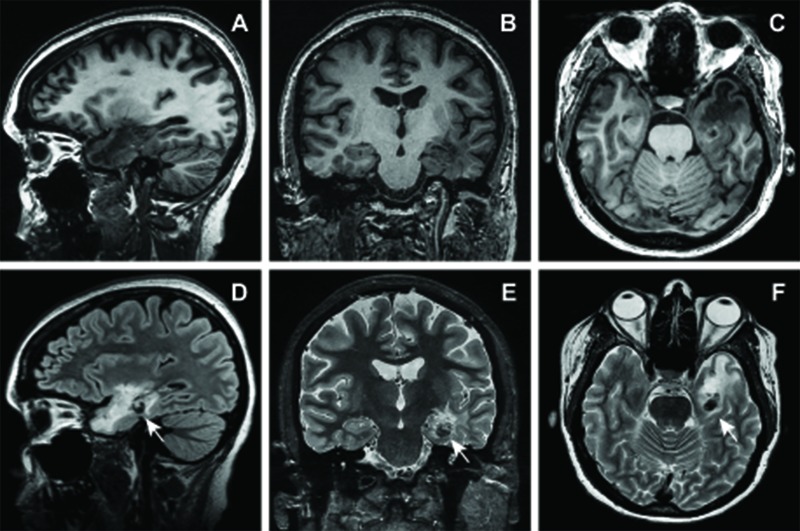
MRI obtained 9.5 years following radiosurgery showing suspected radiation-induced lesion. Sagittal, coronal and axial T1-weighted without contrast (A-C) and T2-weighted (D-F) images demonstrating interval development of a multilobular hetereogenous lesion within the left parahippocampal gyrus which is T2 hypointense with sublte intrinsic T1 shortening with associated susceptibility. A second small focus with similar imaging characteristics is also seen within the left anterior temporal lobe. Arrows, region of interest.
